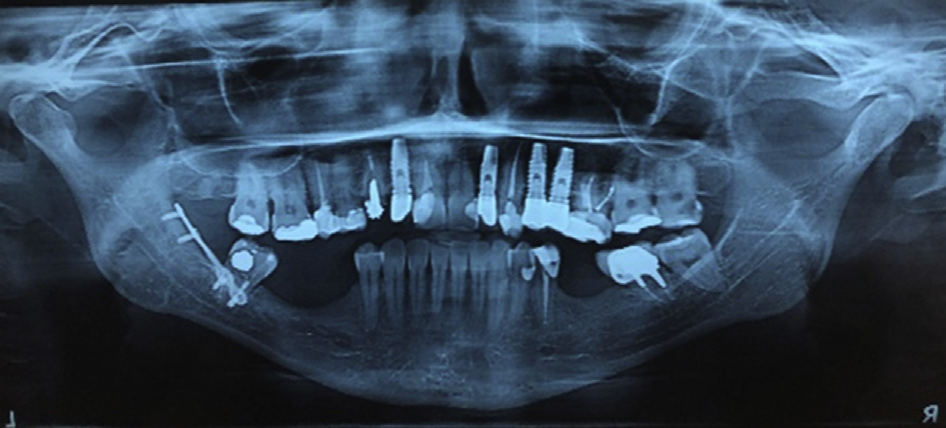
Radiography of 3 years follow up.
